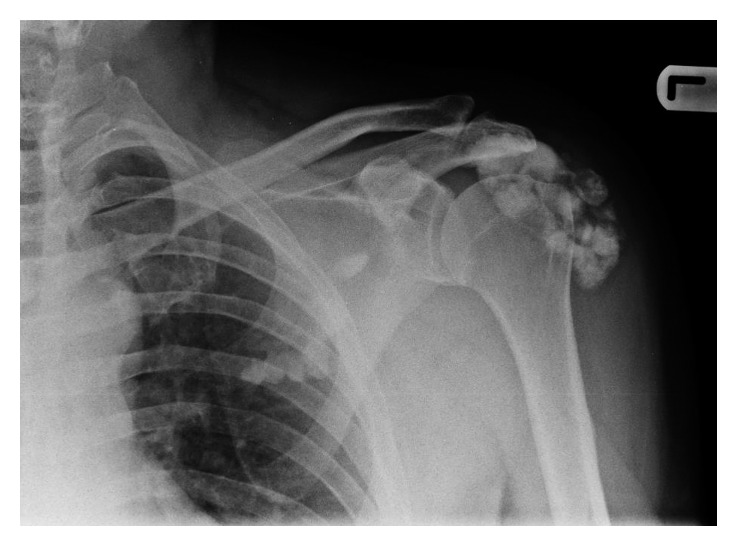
Anteroposterior radiographs of the left shoulder showing calcification over the greater tuberosity and subacromial space as well as classic hyperostotic lesions in the scapular blade.
